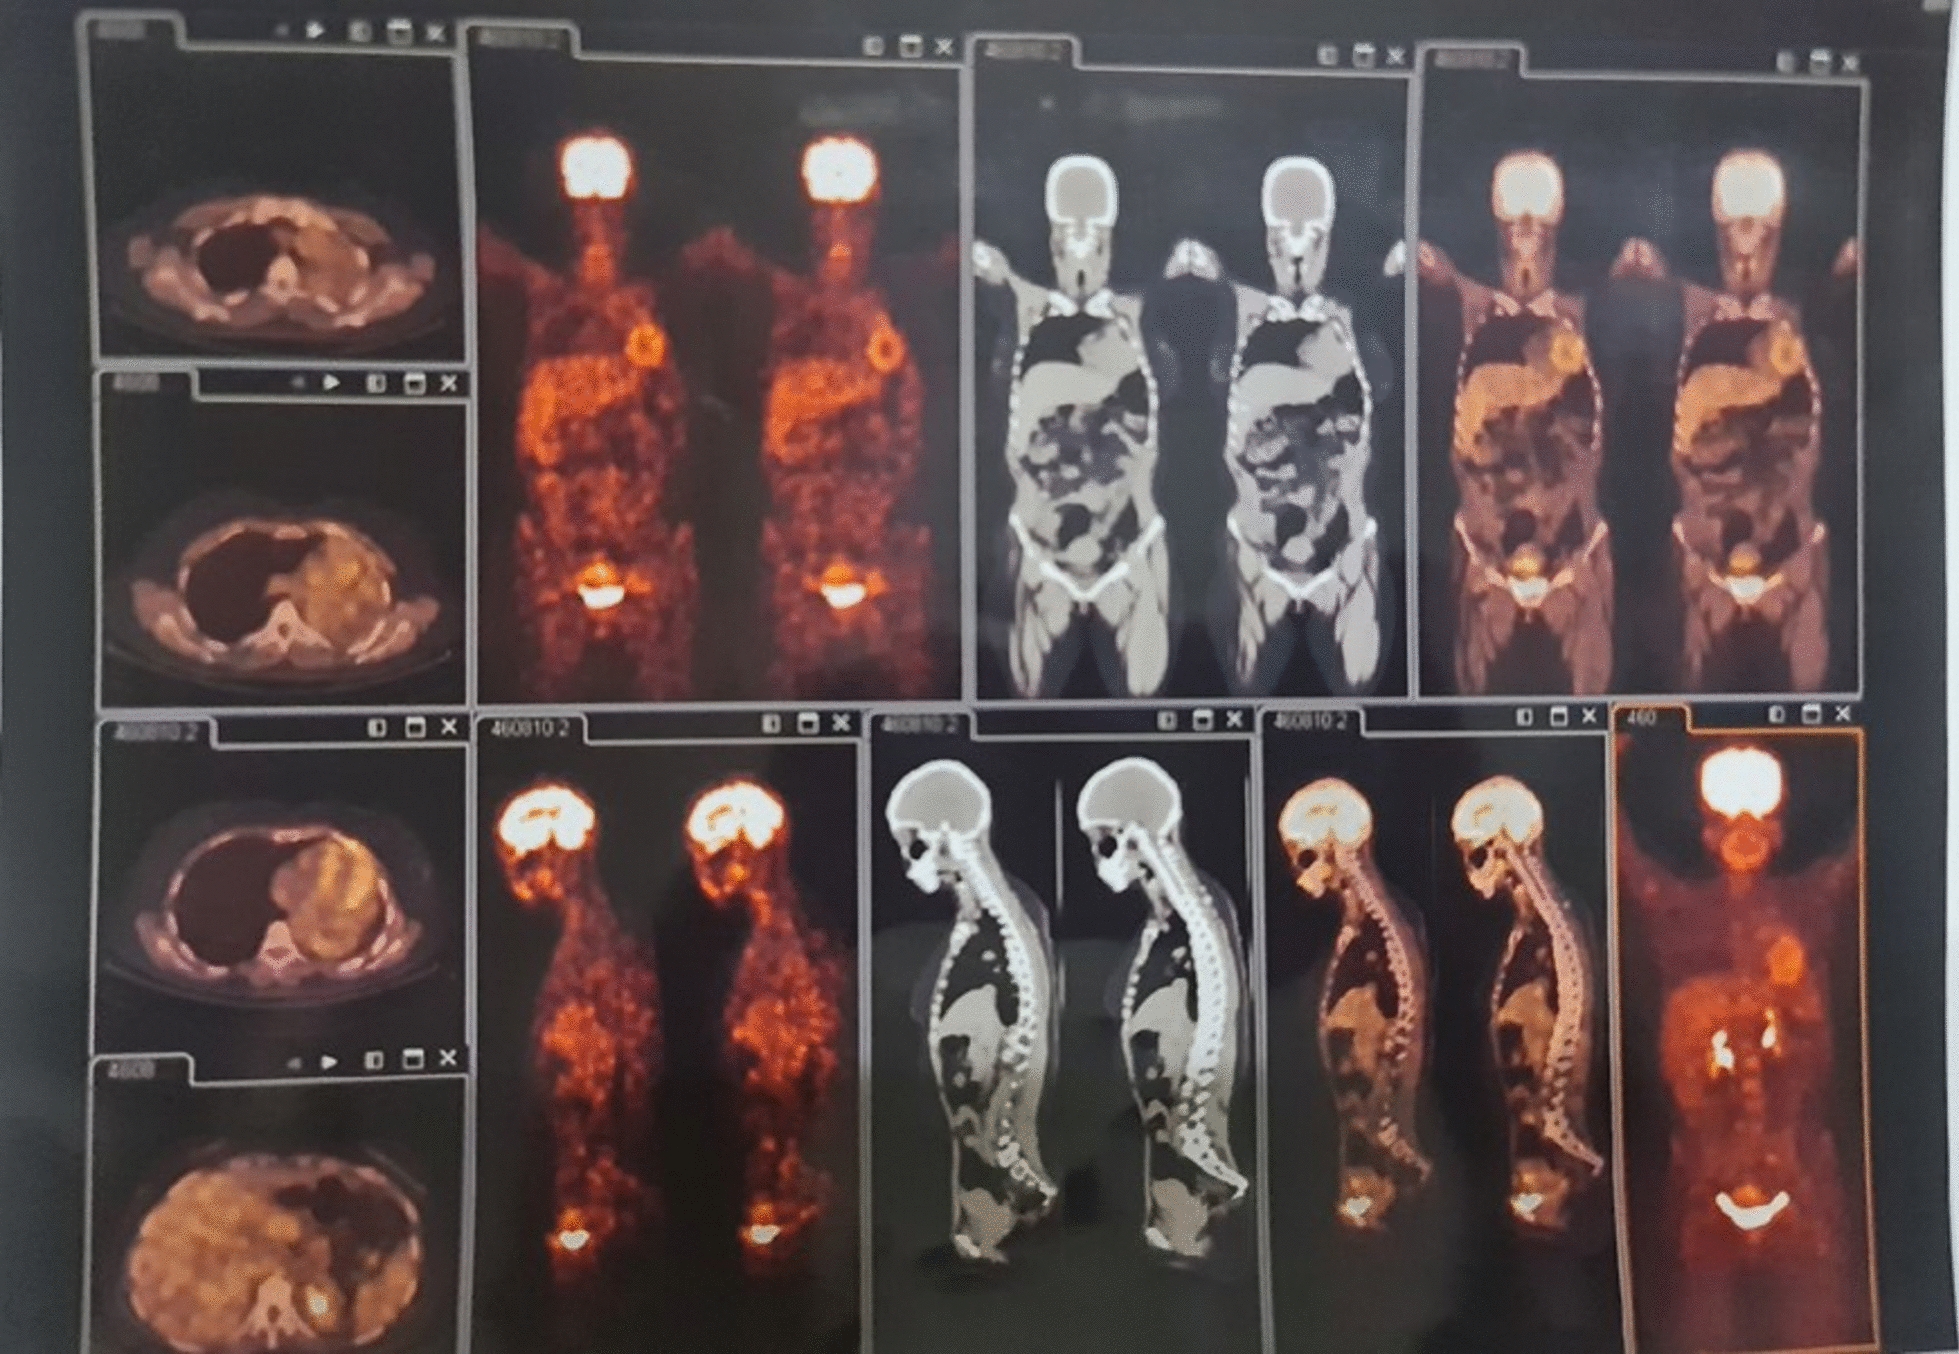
A positron emission tomography–computed tomography showed a complete response to tyrosine kinase inhibitors in a patient with recurrent lung adenocarcinoma. The scan shows left lung resected with fibrosis in its place
